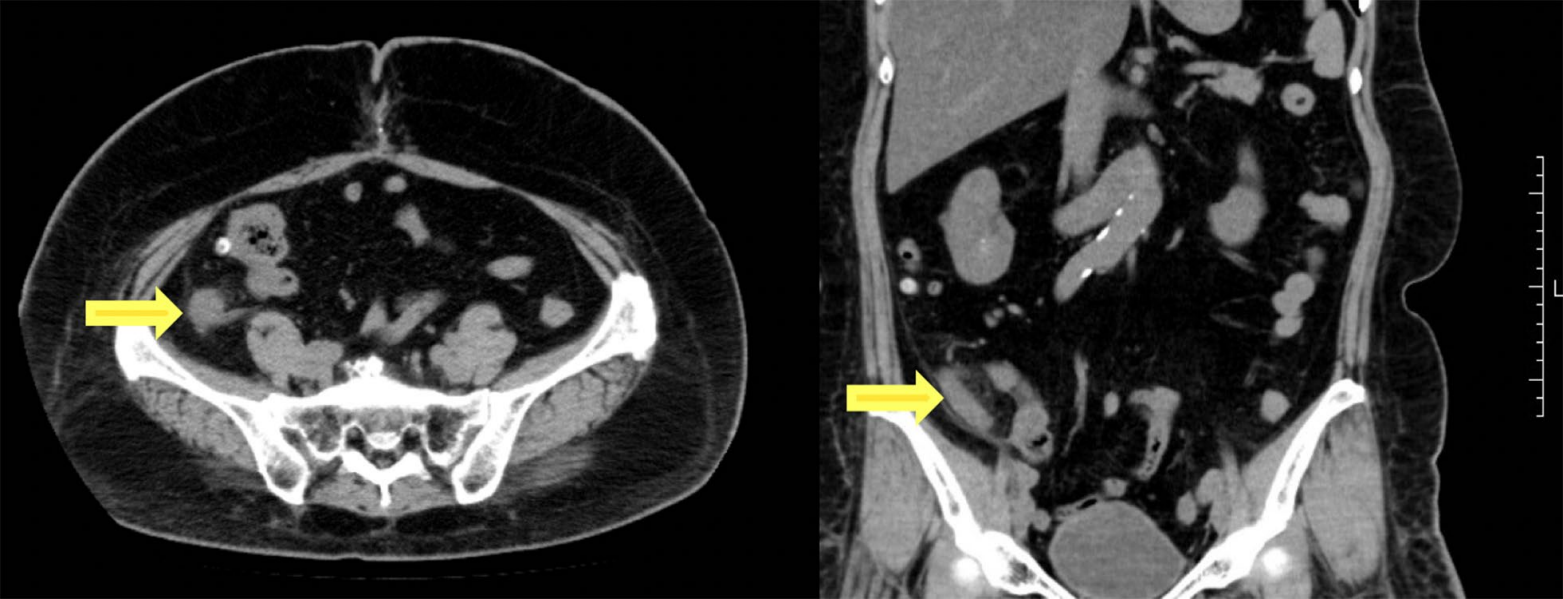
Preoperative computed tomography scan. Contrast-enhanced abdominal computed tomography scan in a 68-year-old woman who presented with nausea and gradually worsening right lower quadrant abdominal pain. The appendiceal diameter was enlarged 14 mm (yellow arrow) and periappendiceal fat stranding was observed
